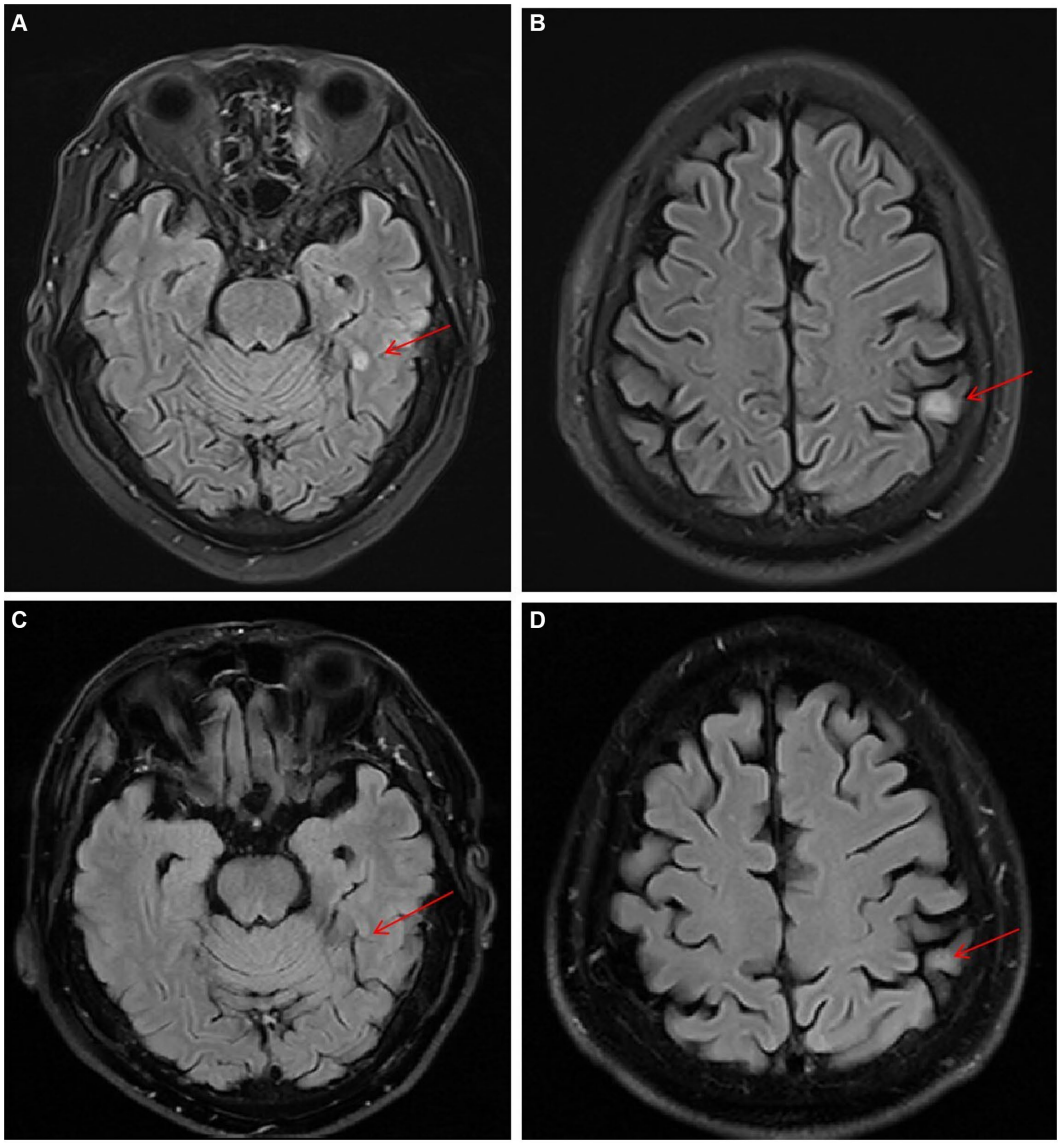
Serial T2-weighted FLAIR MRI images of lesions in the left temporal and parietal lobes (the red arrows). (A,B) Day 32. (C,D) Day171.
